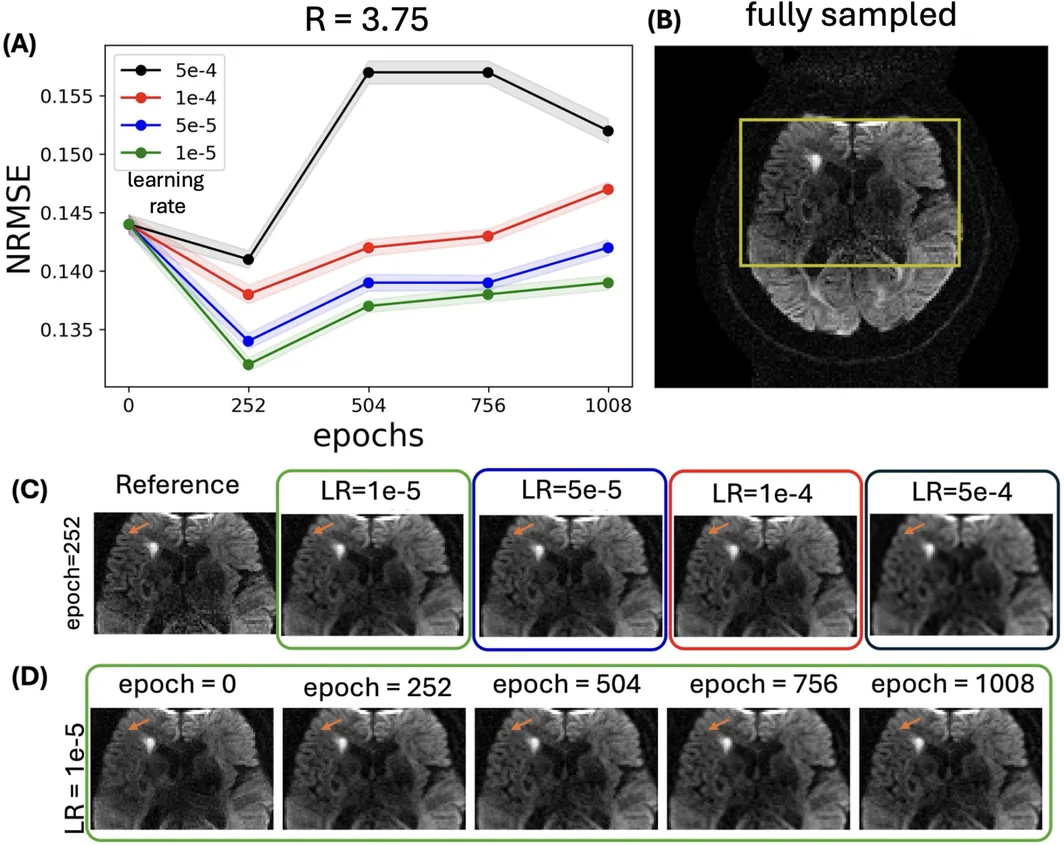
(A) NRMSE of DWI reconstructions averaged over five validation subjects from data retrospectively accelerated by R≈3.75. (B) Fully‐sampled reference. (C) Zoomed‐in reconstructions obtained with fixed epochs and varying learning rate and (D) fixed learning rate and varying epochs. Similar to the fastMRI FLAIR experiments, insufficient or excessive fine‐tuning duration and higher learning rates consistently result in worse reconstructions, demonstrating that the proposed training strategy is effective for clinical stroke DWI data.
